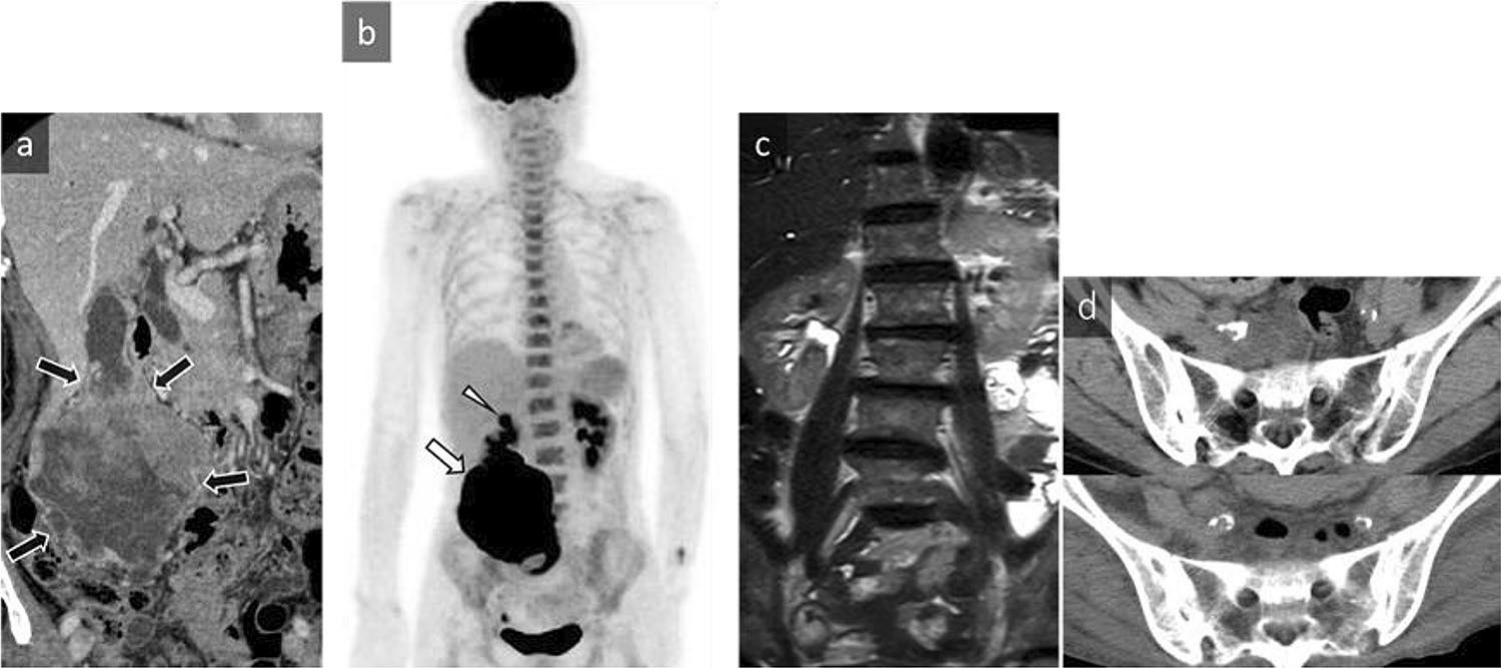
A 79-year-old woman with G-CSF-producing gallbladder carcinoma. a Coronal contrast-enhanced CT shows a 9.5-cm exophytic mass in the fundus of the gallbladder with geographic hypoattenuation and enhancement in the peripheral component (black arrow). b An ^18^F-FDG-PET/CT maximum intensity projection image demonstrates marked FDG uptake in the gallbladder tumor (maximum standardized uptake value = 48.7, white arrow) and gallbladder neck lymph node (white arrowhead), with diffuse uptake in the bone marrow of the spine and pelvis. The splenic uptake appears more intense than the hepatic uptake. c The signal intensity of bone marrow on T2-weighted (half Fourier acquisition single shot turbo spin echo [HASTE]) coronal images is lower than that of an age-matched healthy woman. d Axial CT scans of bone marrow in the spine and pelvic regions on admission (top panel, serum white blood cell count: 15,200) and 3 months after admission (bottom panel, serum white blood cell count: 59,800). CT attenuation of the bone marrow increased in parallel with tumor progression and elevated serum WBC count
